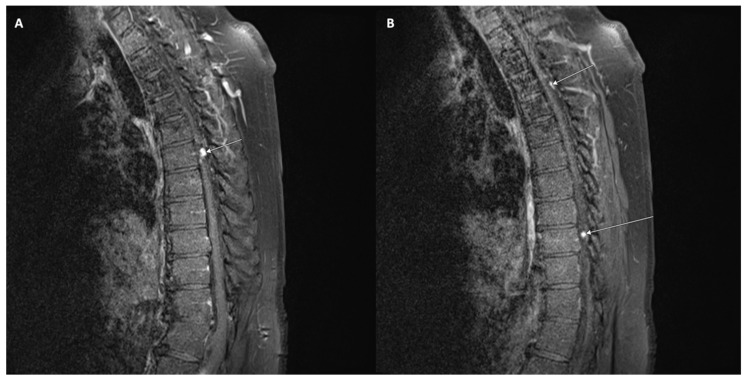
T1-weighted contrast-enhanced MRI demonstrating drop metastases at T6 (A), T3, and T9 (B) in a patient who previously underwent a resection for an ependymoma.
